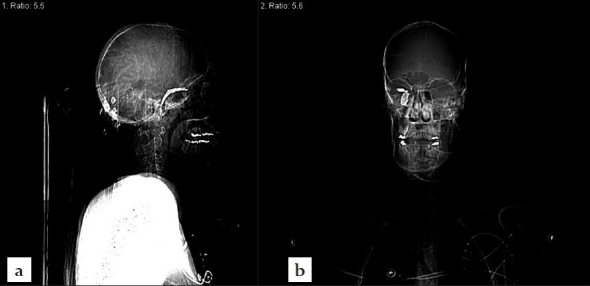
CT scout images demonstrating an intracranially retained foreign body. (a) Lateral view. (b) A/P view.
